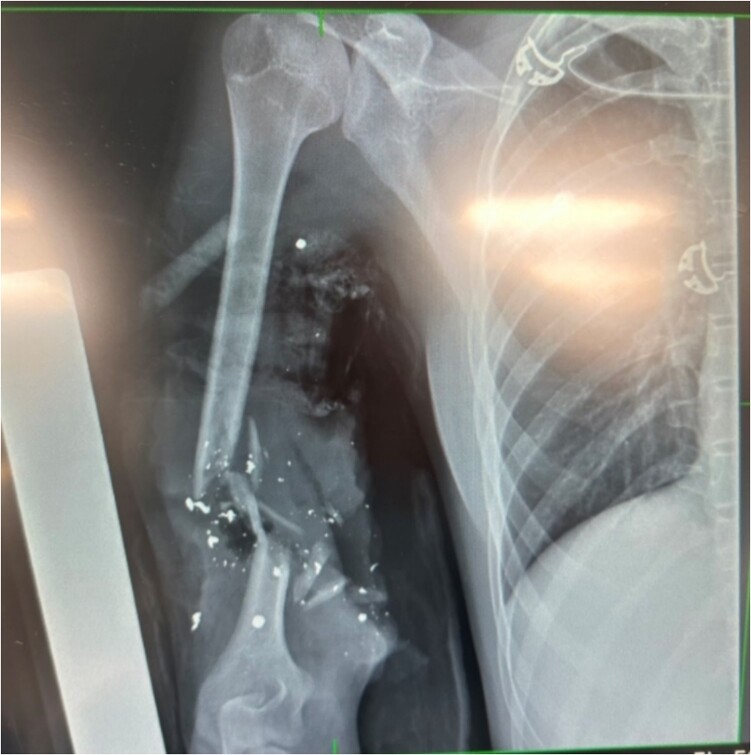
X-ray showing extent of damage to humerus; bullet shrapnel visible.
